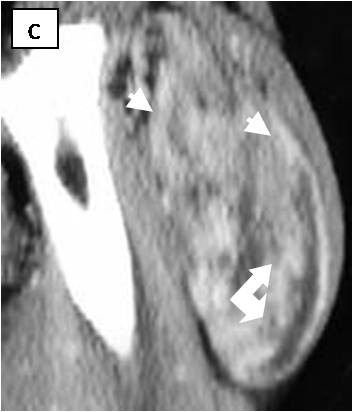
Sagital contrast-enhanced CT scan. Vessels are well visible (white arrows and arrowheads)
